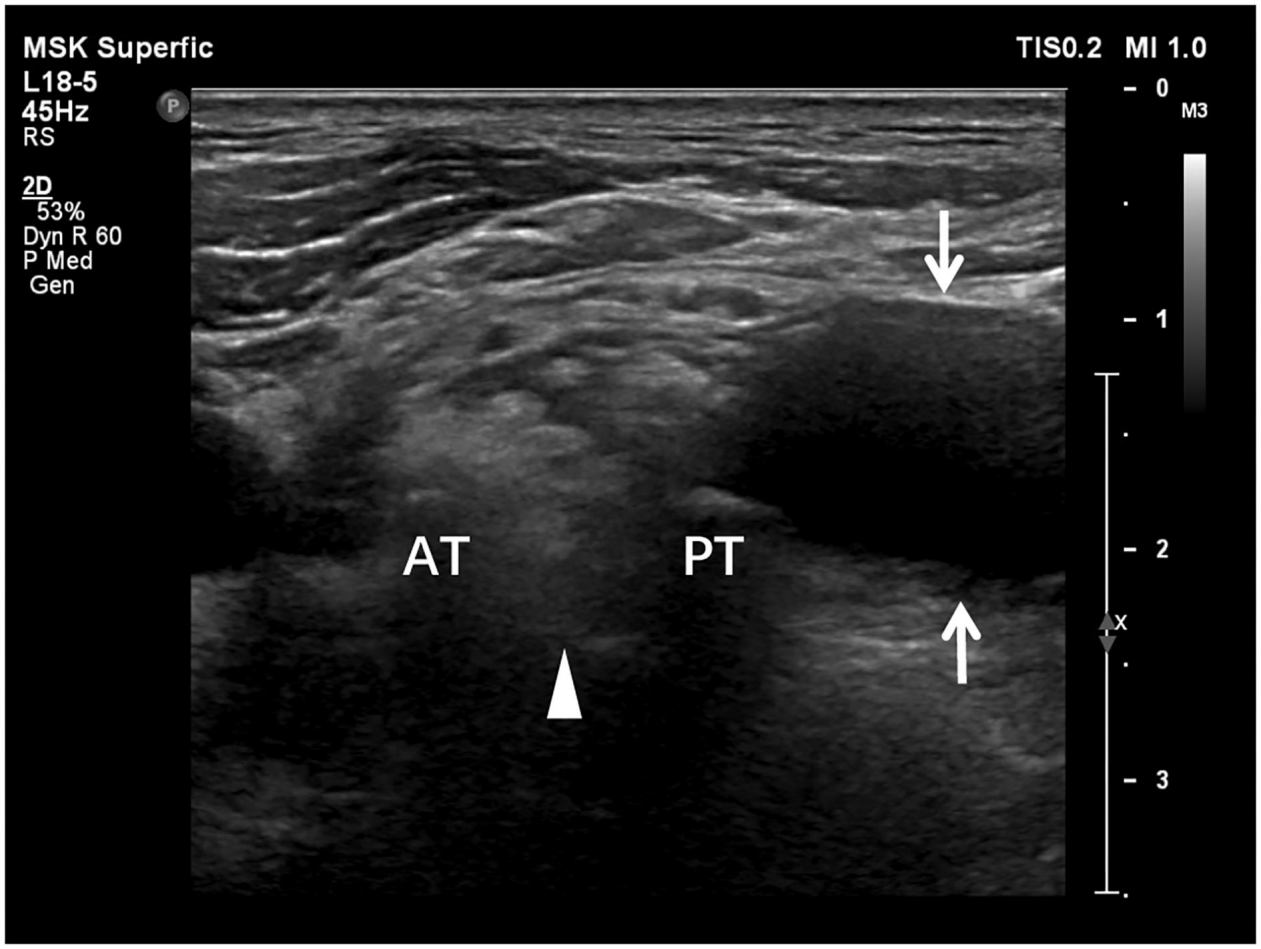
Transverse sonogram shows the accumulated cerebrospinal fluid, i.e., pseudomeningocele, adjacent to the intervertebral foramen. The cerebrospinal fluid (arrows) appears anechoic. The absence of brachial plexus root (arrowhead) between anterior (AT) and posterior tubercles (PT) represents preganglionic root avulsion.
